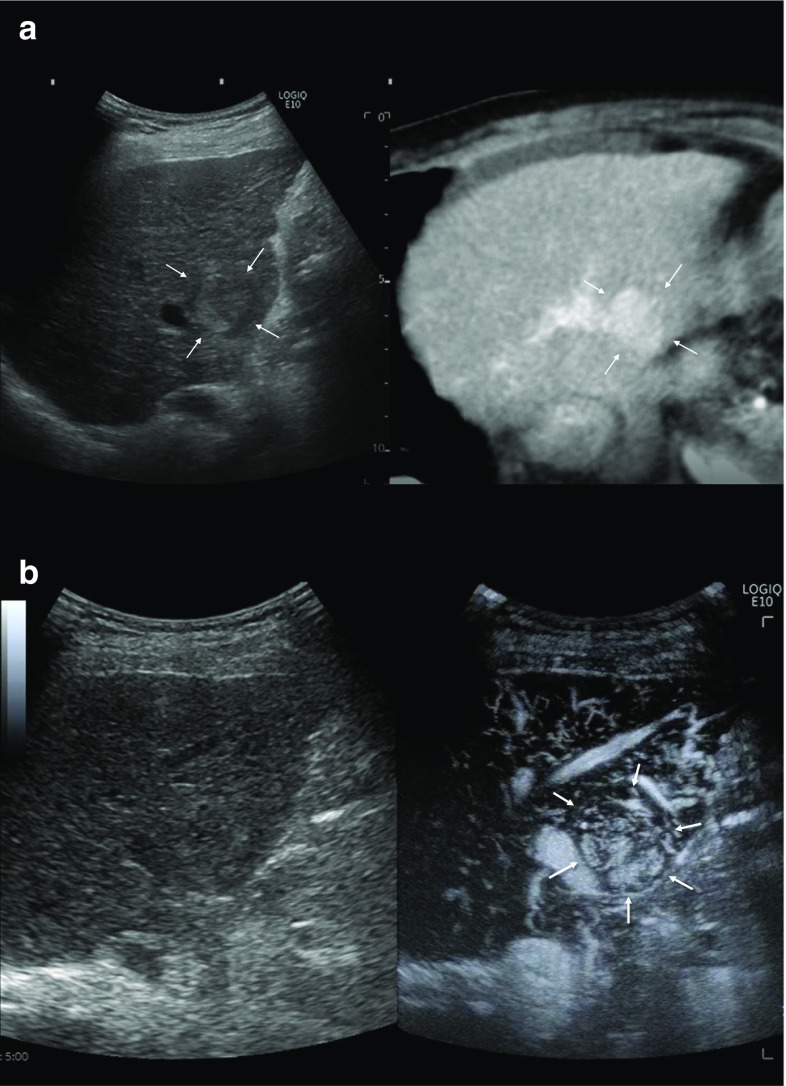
A case of newly developed HCC (maximum diameter 21 mm) in Segment 5. An isoechoic tumor was found at the same site as the contrast-enhanced CT image in the arterial phase using fusion imaging (a). Maximum intensity projection (MIP) can help visualize the fine vascular structure with the superior vessel continuity (b). The arrow points to the location of the identified isoechoic tumor on imaging
